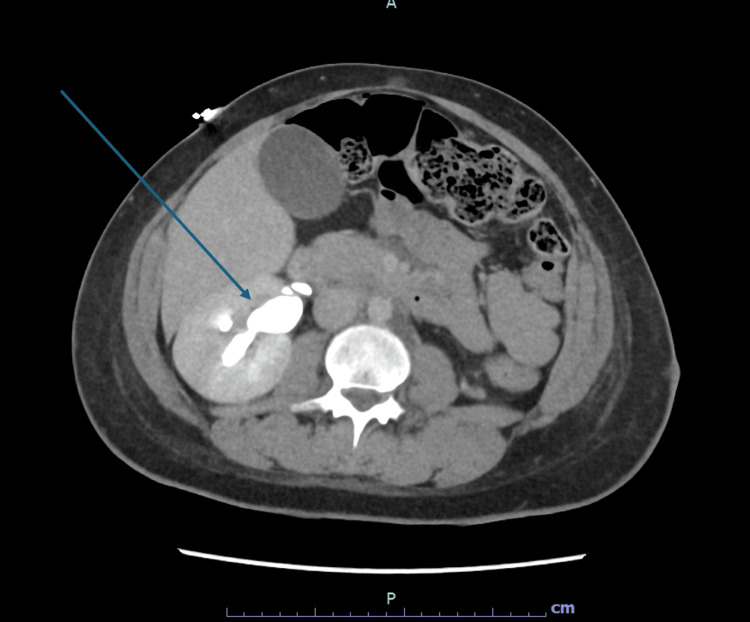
Axial CT intravenous pyelogram demonstrating a hypertrophied right kidney with a tortuous contrast-opacified right ureter coursing around the renal outline (blue arrow). This highlights the complex anatomy of the patient’s solitary functioning urinary tract, relevant to the need for careful right ureteric identification and ureterolysis during haemorrhage control.
